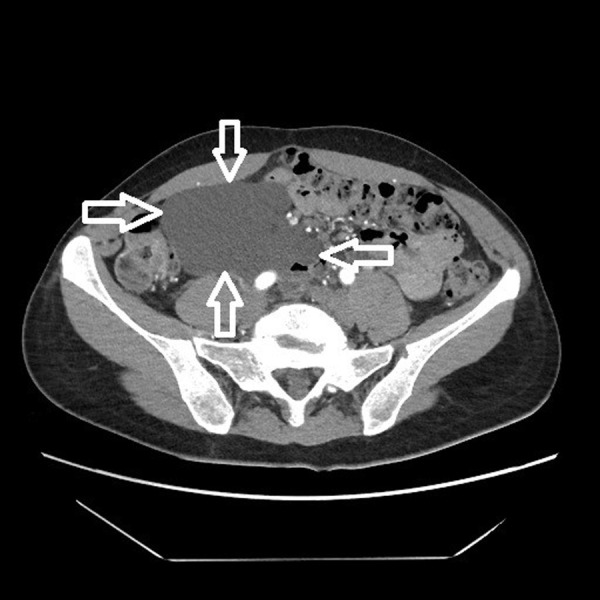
CT scan of the lymphangioma (marked by open arrows) in right abdomen area. The mass is reaching the right side of abdomen wall, contacting abdominal aorta, vena cava and right common iliac artery, also ileocolic artery and vein.
